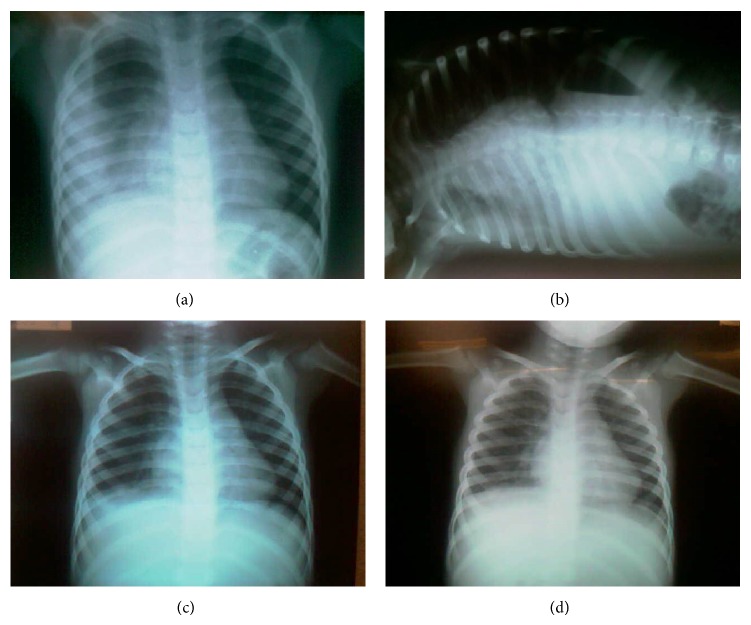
(a) Chest radiograph on the anteroposterior incidence, on day 1 of hospitalization and 15th day of clinical symptoms of pneumonia, showing the lobar pneumonia and a beginning of atelectasis in the right hemithorax. (b) Chest radiography on the incidence of Laurell on 1st day of admission showing pleural effusion in the right hemithorax. (c) Chest radiography on the posterior-anterior incidence on the 4th day of hospitalization, showing atelectasis improvement. (d) Chest radiography on the posterior-anterior incidence on the last day of hospitalization showing atelectasis reversal.
